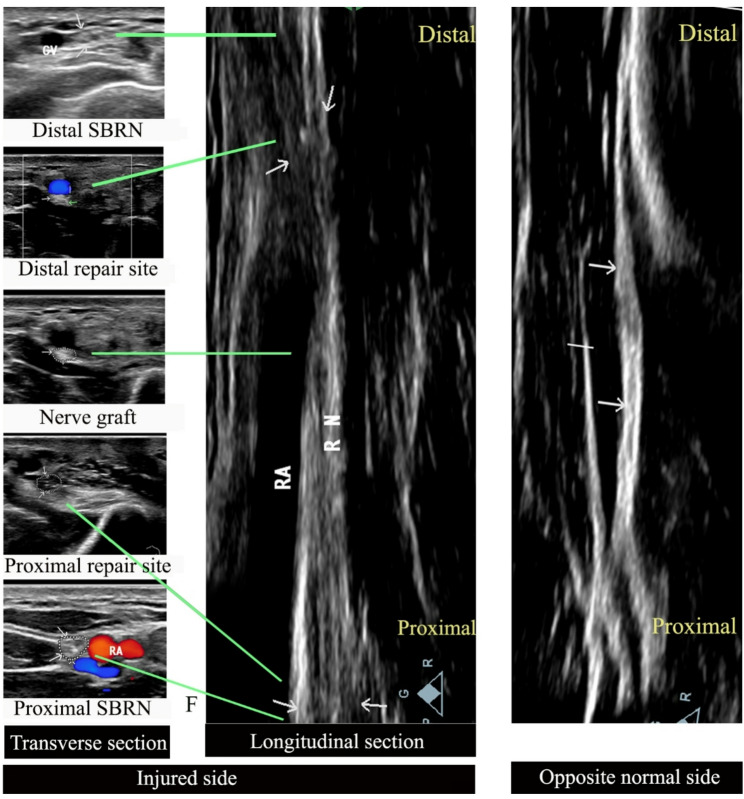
Ultrasonic images of the SBRN (also RN, arrows) at the final follow-up. The green lines show the levels of transverse sections corresponding to the axial section of the SBRN. RA, radial artery. CV, cephalic vein
